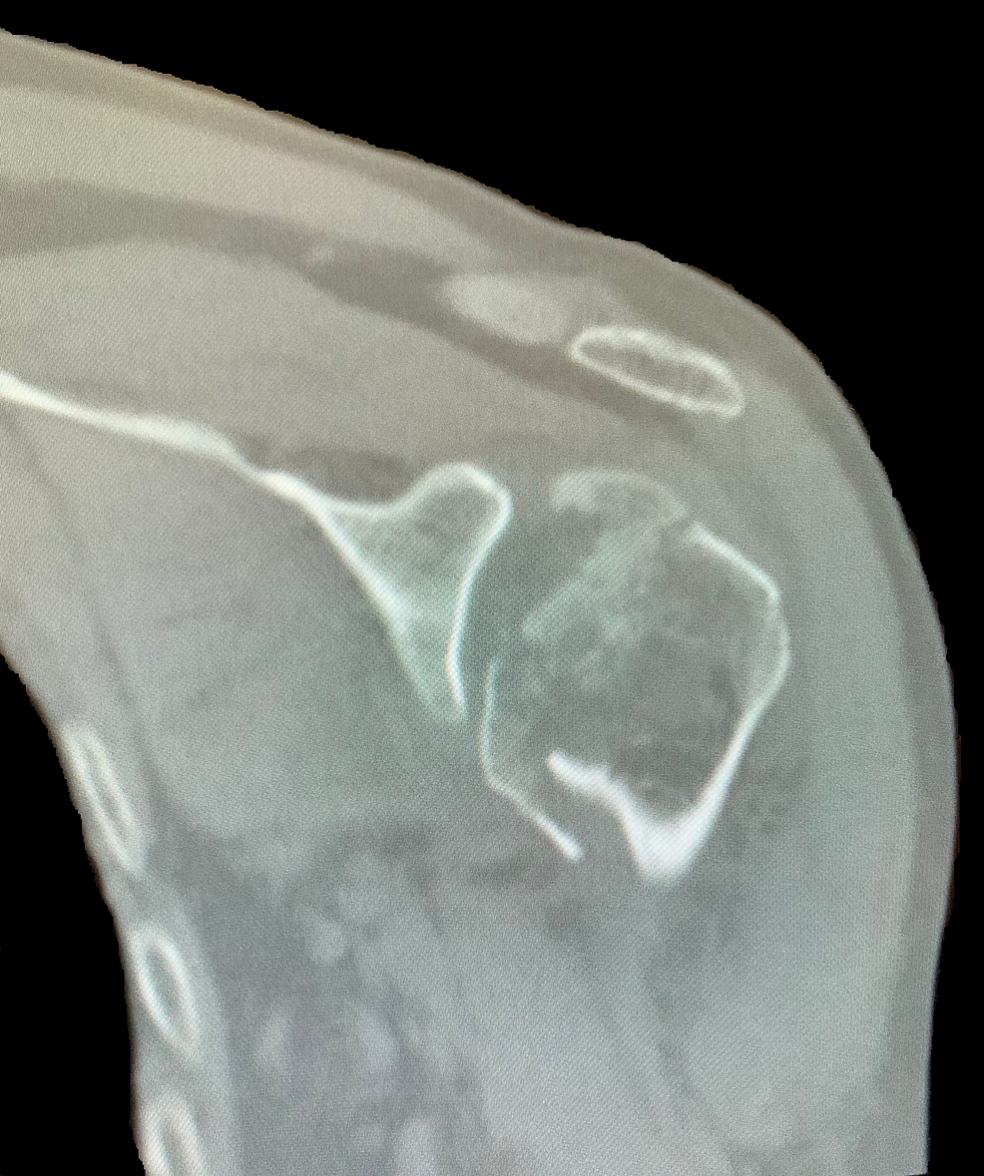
Coronal view of preoperative CT scan of the left shoulder demonstrating a four-part proximal humerus (PH) posterior fracture-dislocation.
